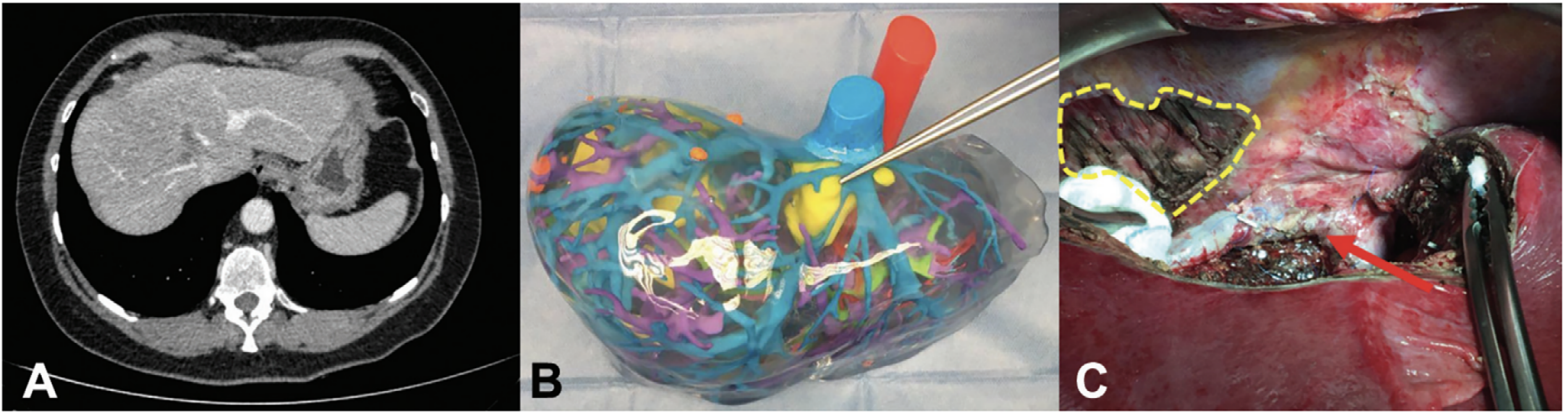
3D printing‐guided liver surgery. A) Preoperative CT scan of a patient with colorectal liver metastases. B) 3D printing of a liver model relative to the patient. C) Post‐resection reconstruction of the right hepatic vein with a peritoneal patch (indicated by the red arrow) taken from the diaphragm (highlighted by the yellow line) (Reproduced with permission). Copyright 2021, Lippincott Williams and Wilkins Ltd.^[^
